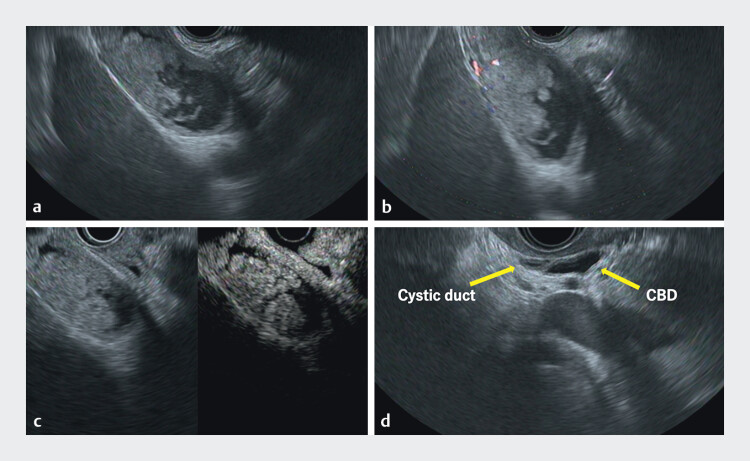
Endoscopic ultrasound images.
